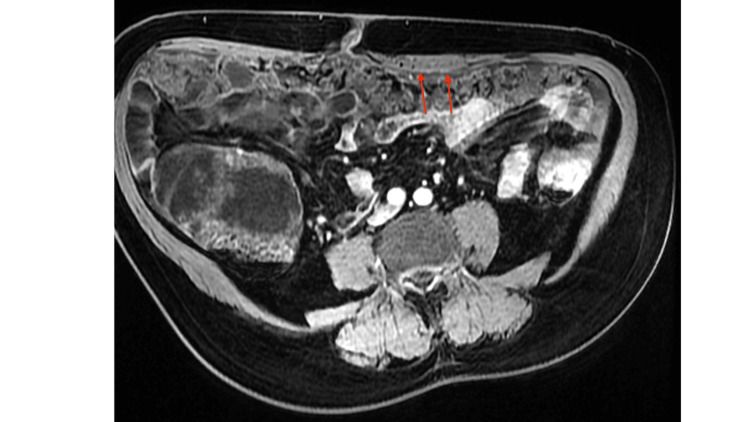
Axial MRI in lava sequence, showing omentum thickening Thickening denoted by the red arrows, suggestive of infiltration of the omental fat.
